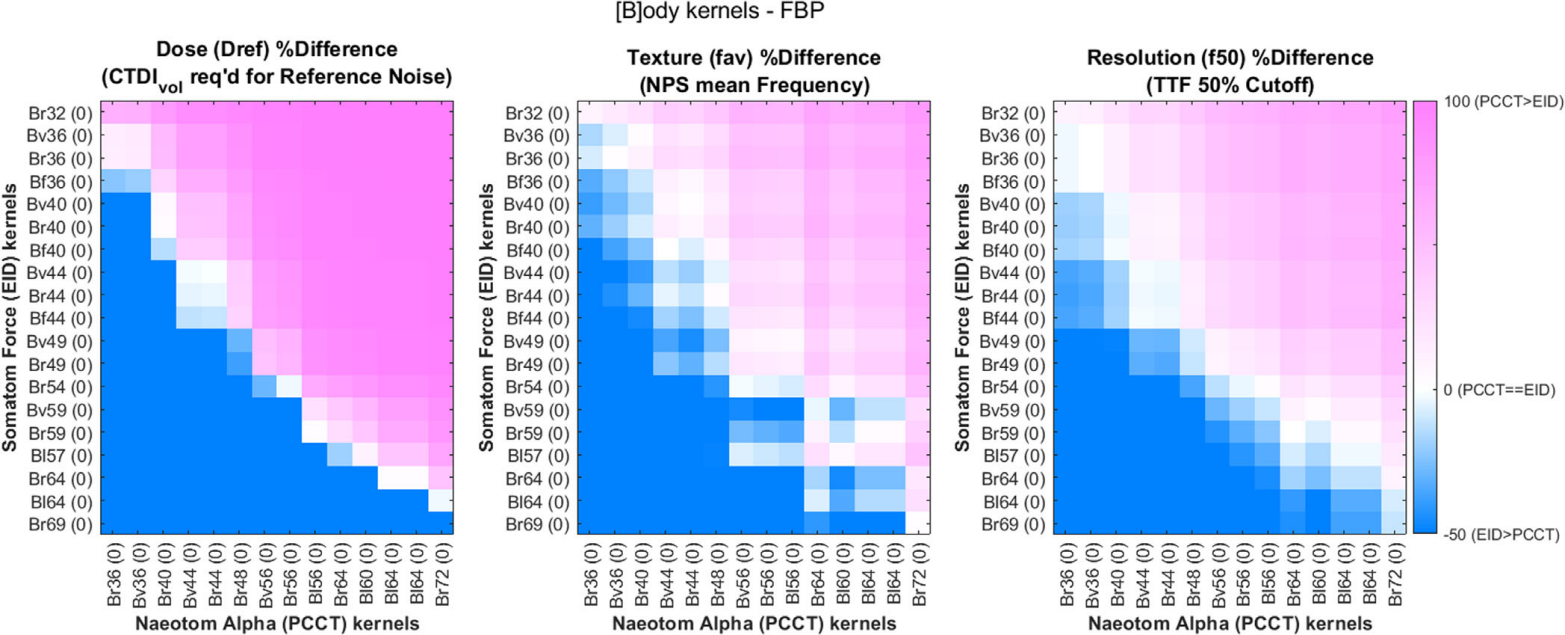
Relative differences in image perception metrics between all Body kernels with filtered back‐projection reconstruction on EID and PCCT systems, computed relative to EID kernels. FBP and IR results can be found in supplemental figures. Both axes are sorted by reference Dose (dref) in all plots for ease of presentation. Head and quantitative kernels showed similar results and can be found in supplemental figures. EID, energy integrating detectors; PCCT, Photon‐counting computed tomography; IR, Iterative Reconstruction.
